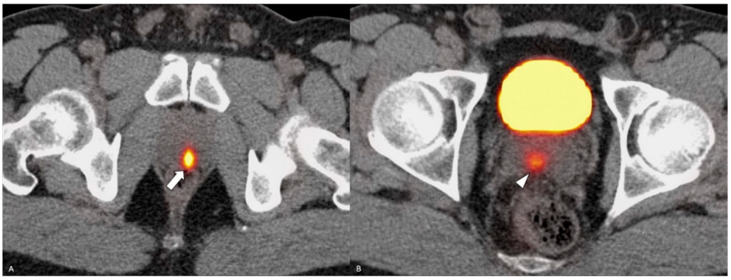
PSMA PET/CT. Part (A)—radiotracer uptake is demonstrated in the posterior prostate (white arrow). Part (B)—tumor is extending into the seminal vesicles (white arrowhead).
